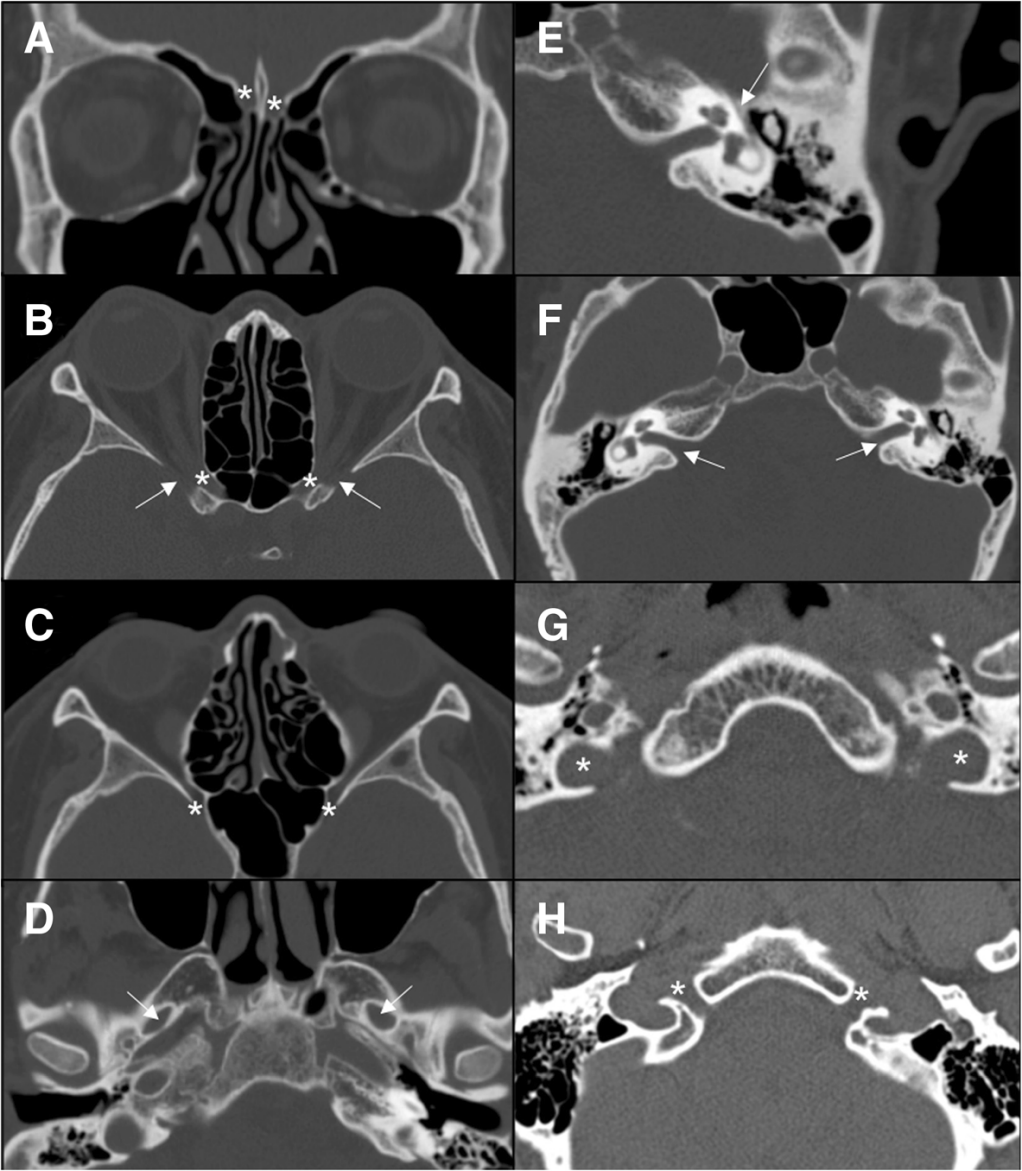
CT aspect of the most important foramina of the skull base. a Cribriform plate (asterisk); b optic canal (asterisk) and superior orbital fissure (arrow); c foramen rotundum (asterisk); d foramen ovale (arrow); e facial canal (arrow); f internal auditory meatus (arrow); g jugular foramen (asterisk); h hypoglossal canal (asterisk)
